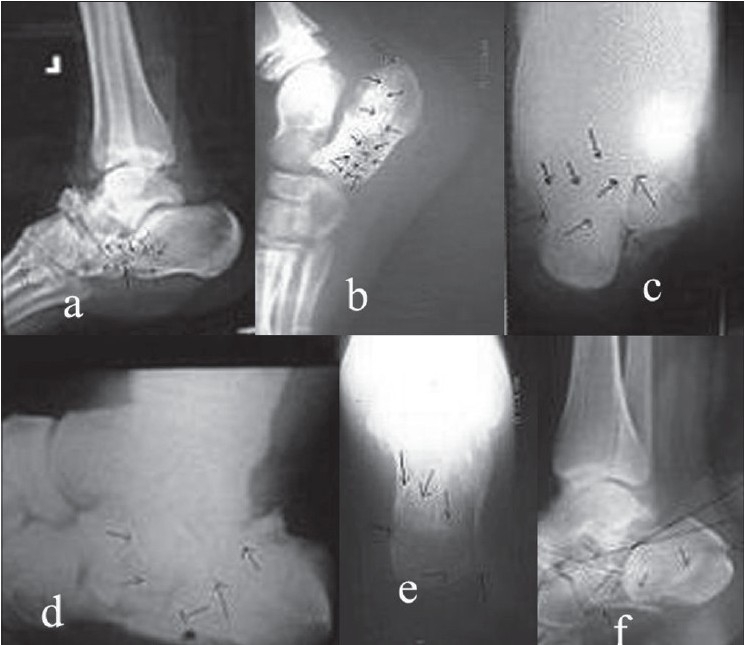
X-rays showing the fracture patterns of secondary fracture line (a) calcaneocuboid type of anterior secondary fracture lines, (b) plantar type of anterior secondary fracture lines (c) Type A of posterior secondary fracture lines pattern, (d) Type B of posterior secondary fracture lines pattern, (e) Type C of posterior secondary fracture lines pattern and (f) Type D of posterior secondary fracture lines pattern
